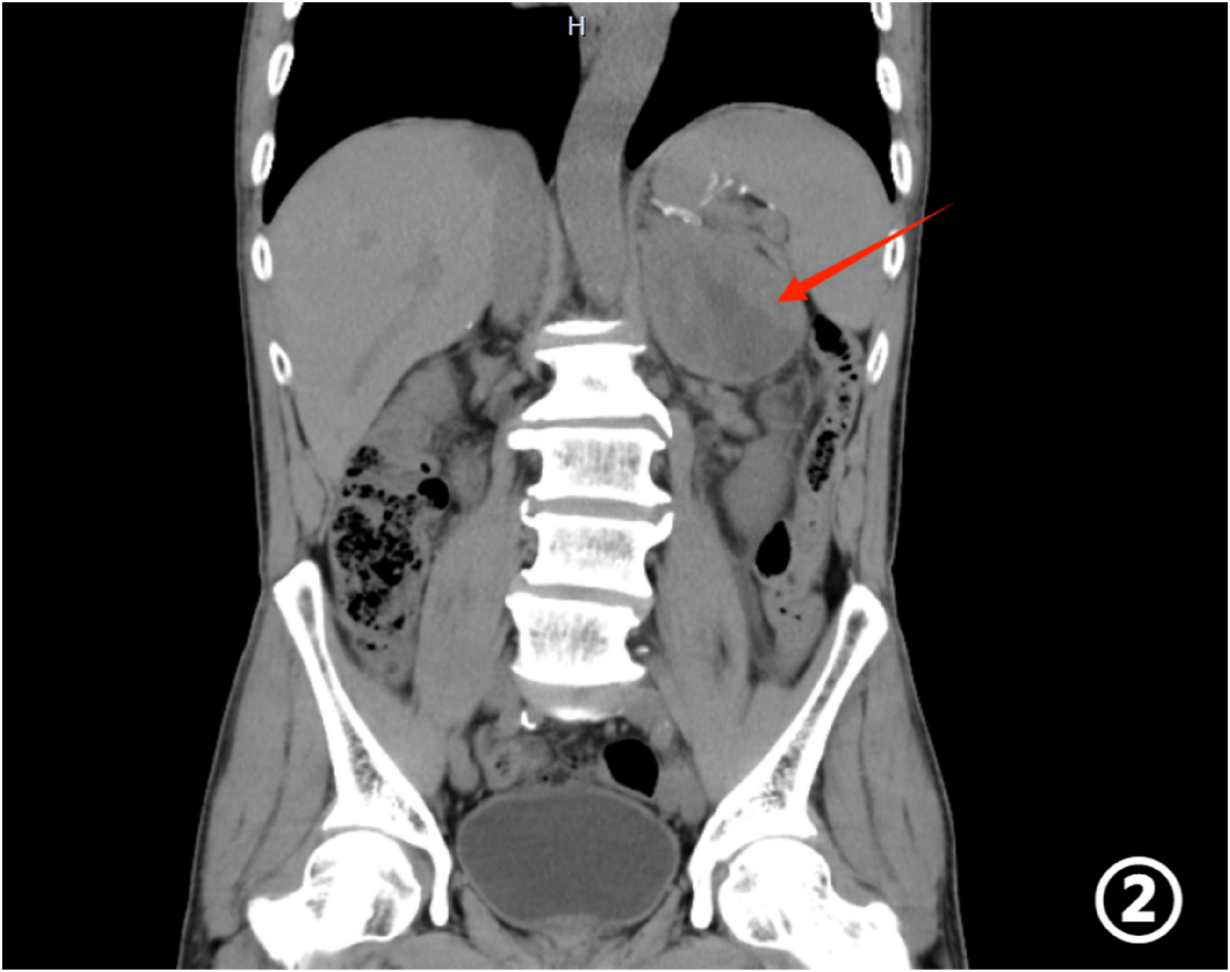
Abdominal CT coronal image: The red arrow points to the spleen area in the left upper abdomen. In the left adrenal region, there is a large soft tissue mass, with multiple nodular shadows visible around it.
